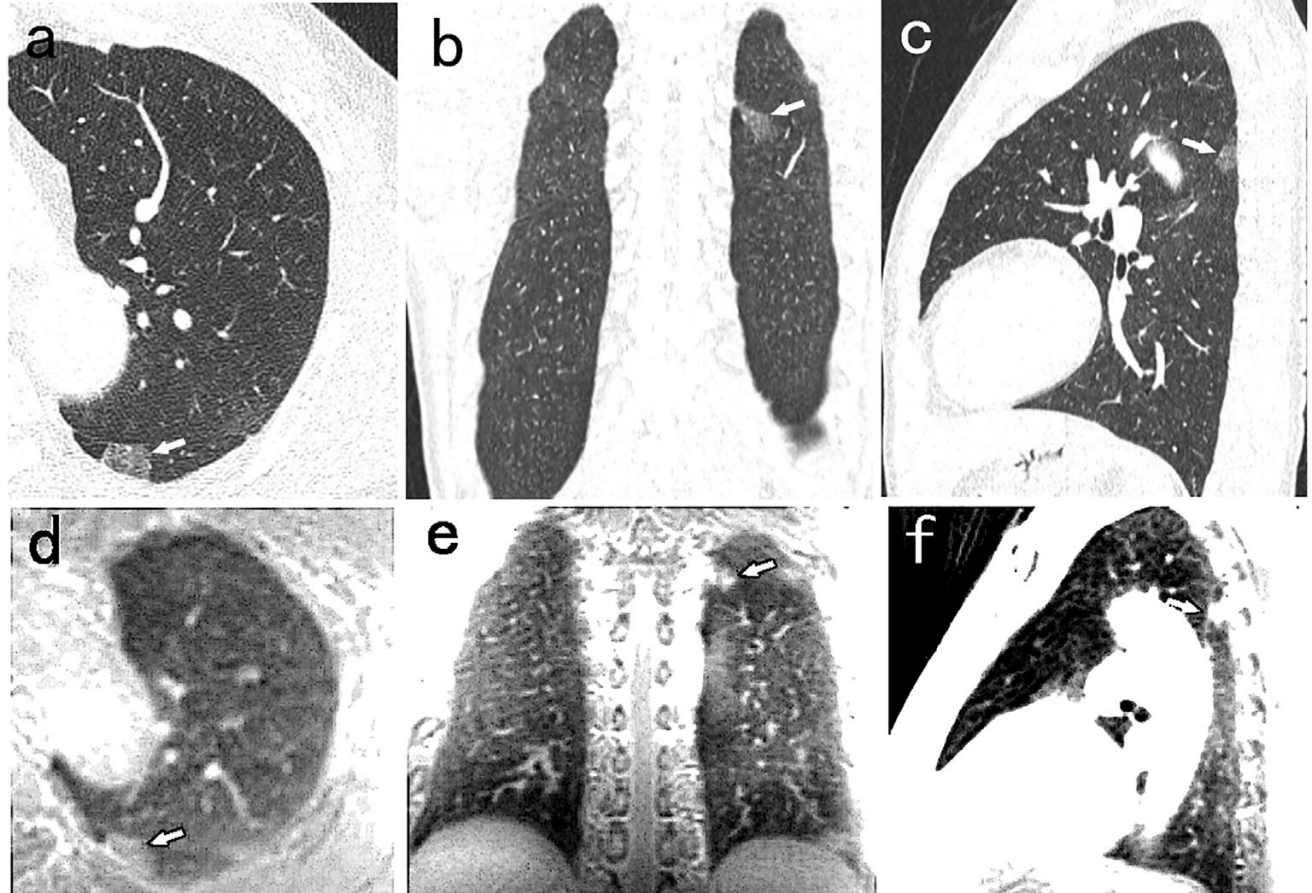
A 52-year-old man with 14mm PSN (arrow) in the left lower lobe. The nodule is well visualized on the CT axial (A), coronal (B), and sagittal (C) image, and on the PETRA sequence axial (D), coronal (E), and sagittal (F) image. The arrows represent the locations of nodules.
